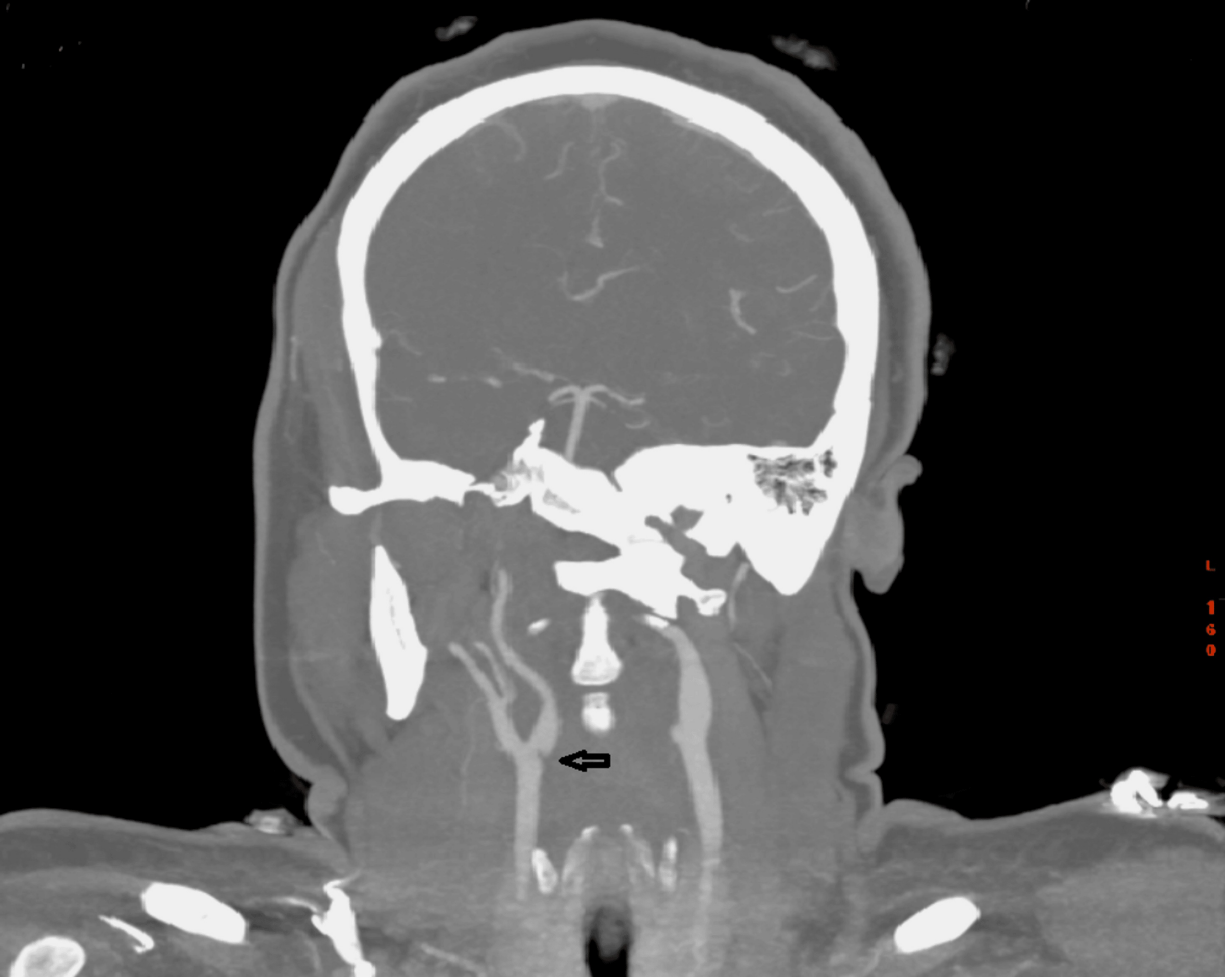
CT carotid angiogram showing a shelf-like structure in the right internal carotid artery (arrow)
